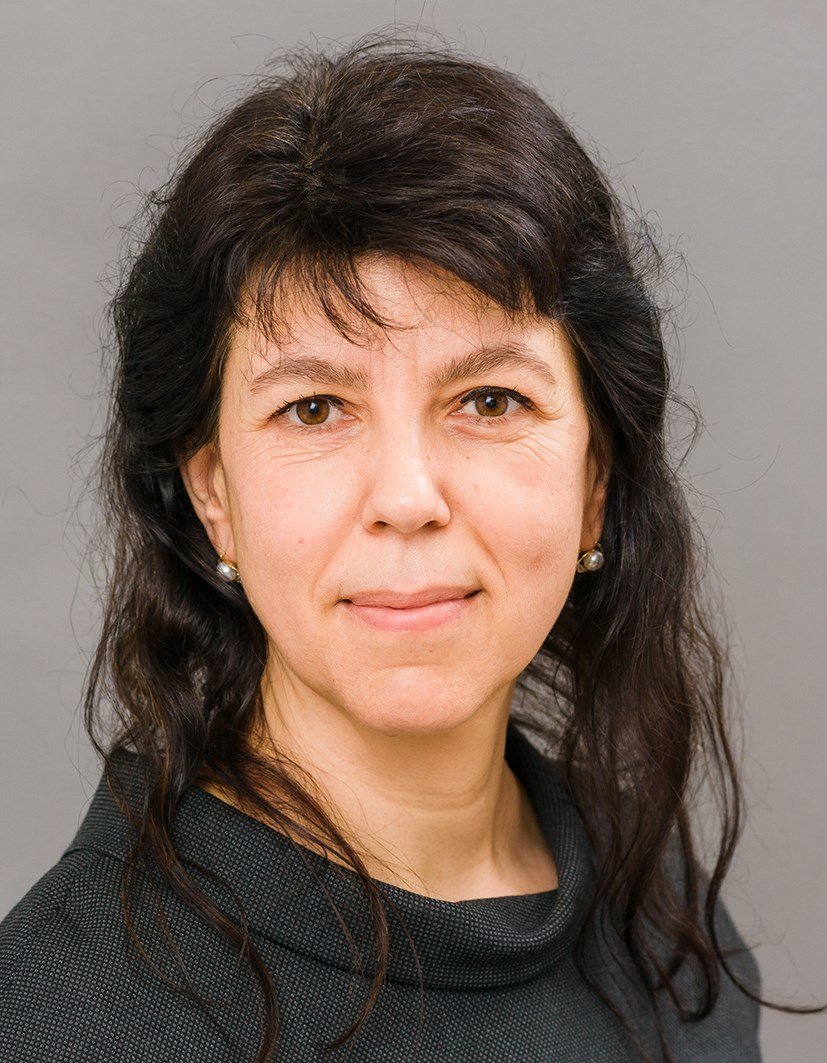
Assoc. Prof. M. Dimova-Mileva is a specialist in Internal Medicine and Cardiology, with additional ESC/EACVI certification in transthoracic echocardiography and expertise in abdominal and vascular ultrasonography.
